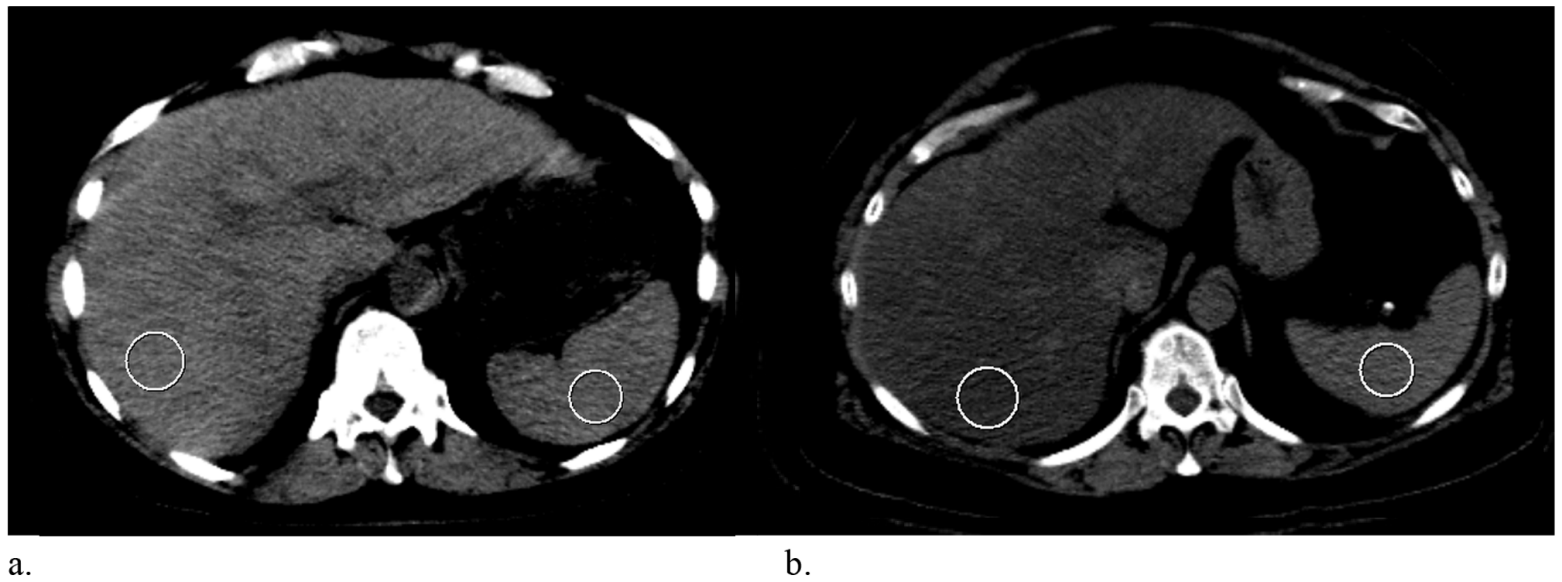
Unenhanced Computed Tomography (CT) scan of the upper abdomen, axial view. Normal liver in a 58-year-old female; liver attenuation is 62 HU, spleen is 55 HU, liver-spleen ratio is 1.1 (a). 64-year-old female with liver steatosis; the liver parenchyma (28 HU) is relatively hypodense compared to the spleen (55 HU), liver-spleen ratio is 0.5 (b). HU: hounsfield units.
